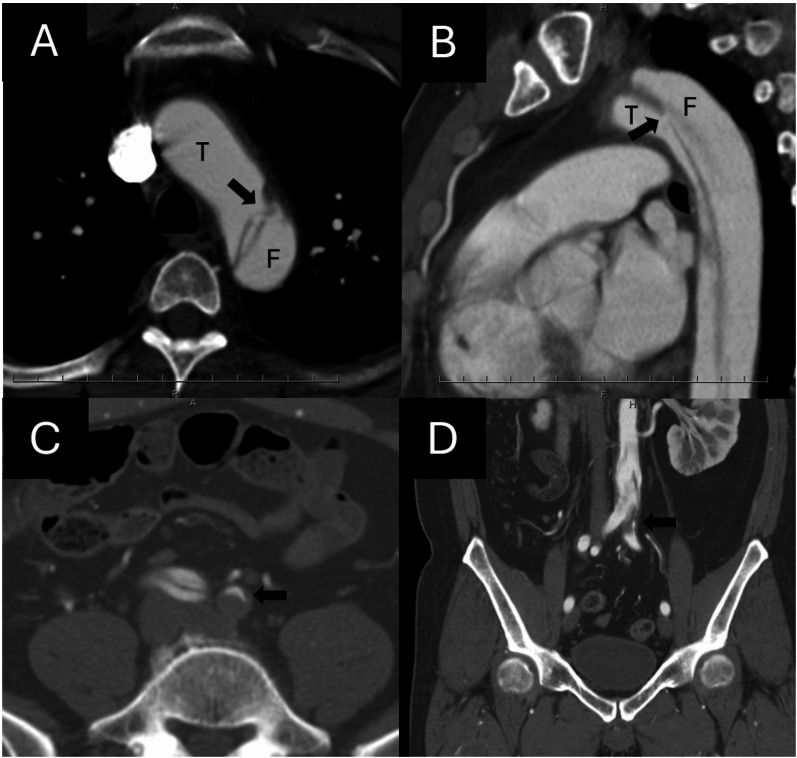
Preoperative contrast-enhanced computed tomography (CT) images. (A, B) Axial and sagittal views at the aortic arch level show the primary entry tear (black arrows) located just distal to the origin of the left subclavian artery (LSA). (C, D) Axial and coronal views at the level of the iliac bifurcation demonstrate collapse of the left common iliac artery (arrows) due to compression of the true lumen by the false lumen
